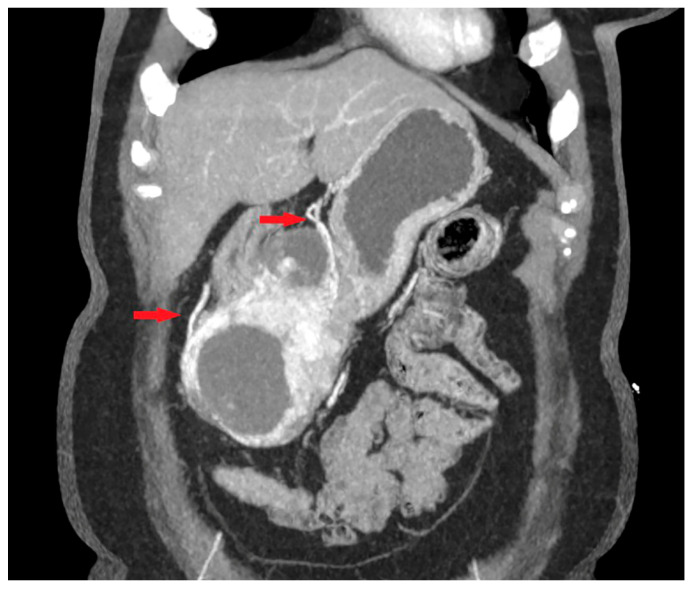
CT coronal view shows irregular, partially cystic tumor with hyperdense solid component and presence of peri- and intra-tumoral vascular vessels (red arrows) (EFDV).
